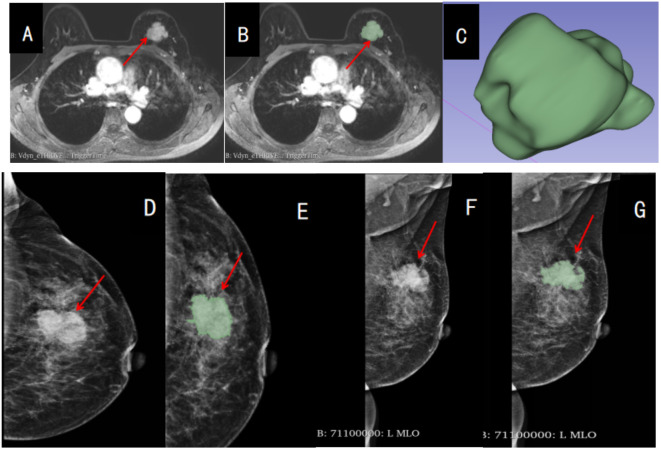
Representative images from a 52-year-old female with left breast invasive carcinoma (HER2-positive). (A–C) Contrast-enhanced T1-weighted MRI with sequential slice-by-slice delineation. (A, B) Axial images show a lobulated, markedly enhancing mass (red arrows). (C) Fused 3D ROI reconstruction. (D, E) CC view mammography: (D) Irregular lobulated mass in the upper central left breast (red arrow); (E) Corresponding ROI delineation. (F, G) MLO view: (F) Same mass as in panel (D) (red arrow); (G) ROI delineation.
